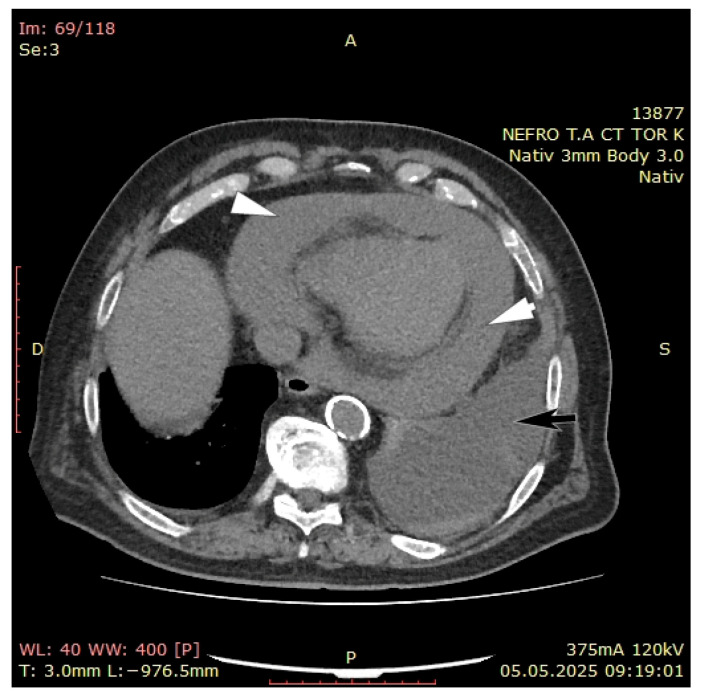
CT with contrast agent revealing important pericardial (white arrows) and pleural effusion (black arrow).
